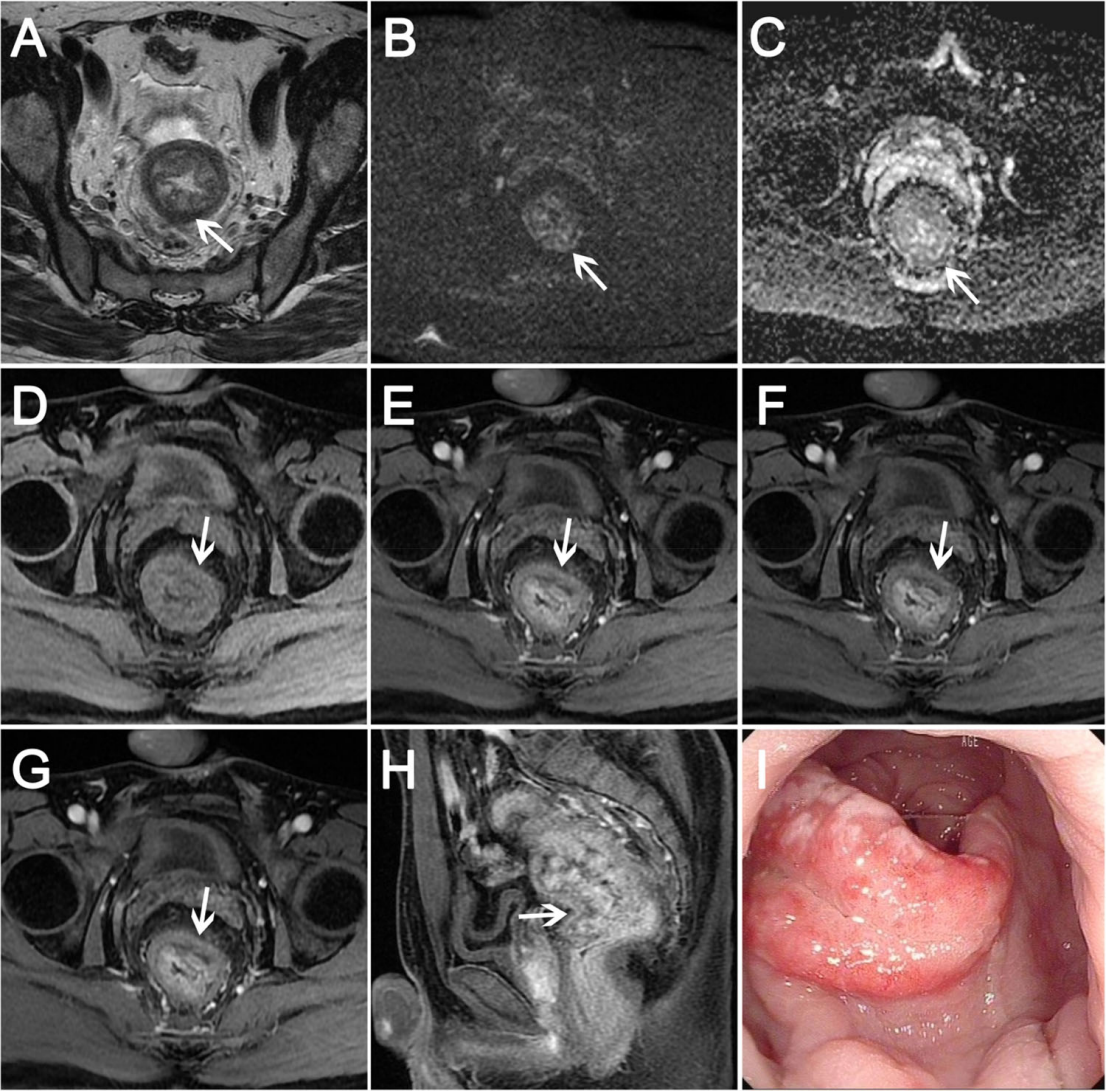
A 35-year-old man presenting with repeated massive prolapse for more than 2 years. A Axial T2WI shows diffuse thickening with slightly hyperintensity. B Unrestricted diffusion was observed on DWI and (C) ADC map. D T1WI shows isointense signal. E–H Persistent and layer enhancement was observed on the multi-phase contrast-enhanced sequences. I Colonoscopy shows circumferential irregular mucosal hyperemia, edema, and surface erosion at 30–160 mm from the anal verge
